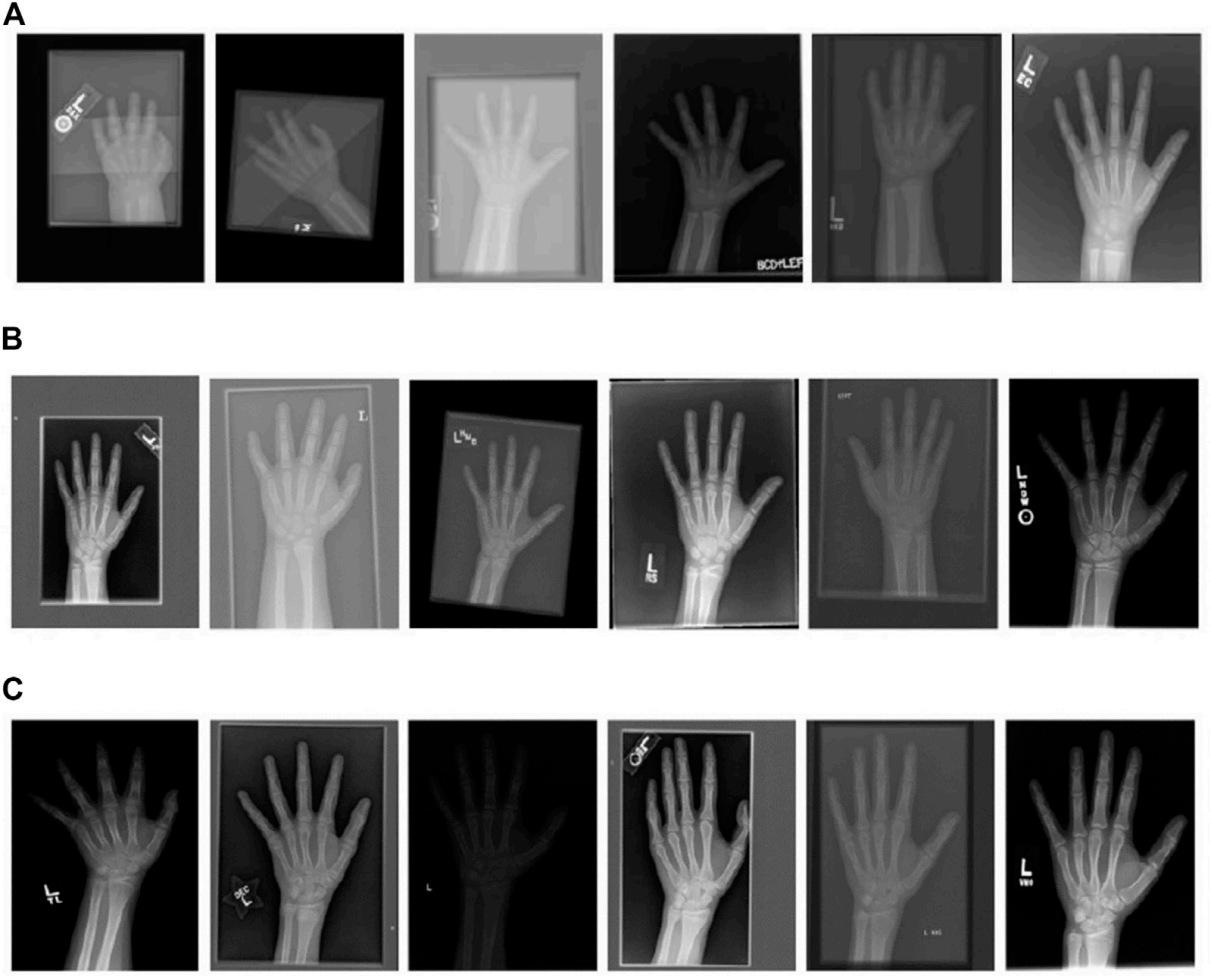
X-rays of hands based on (A) 1–6 years old; (B) 7–12 years old; (C) 13–18 years old.
